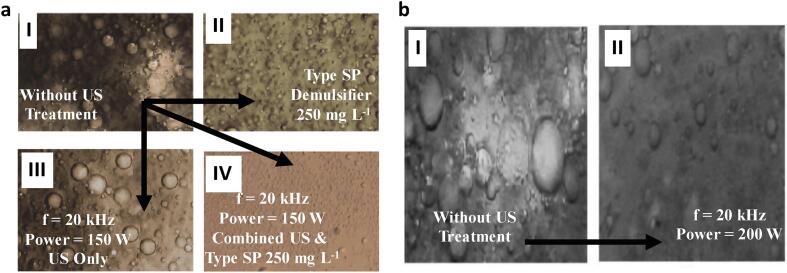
The mechanism of ultrasonic demulsification: a Effect of US with and without chemical demulsifiers [38]b Effect of US on super heavy crude oil emulsion [50].
